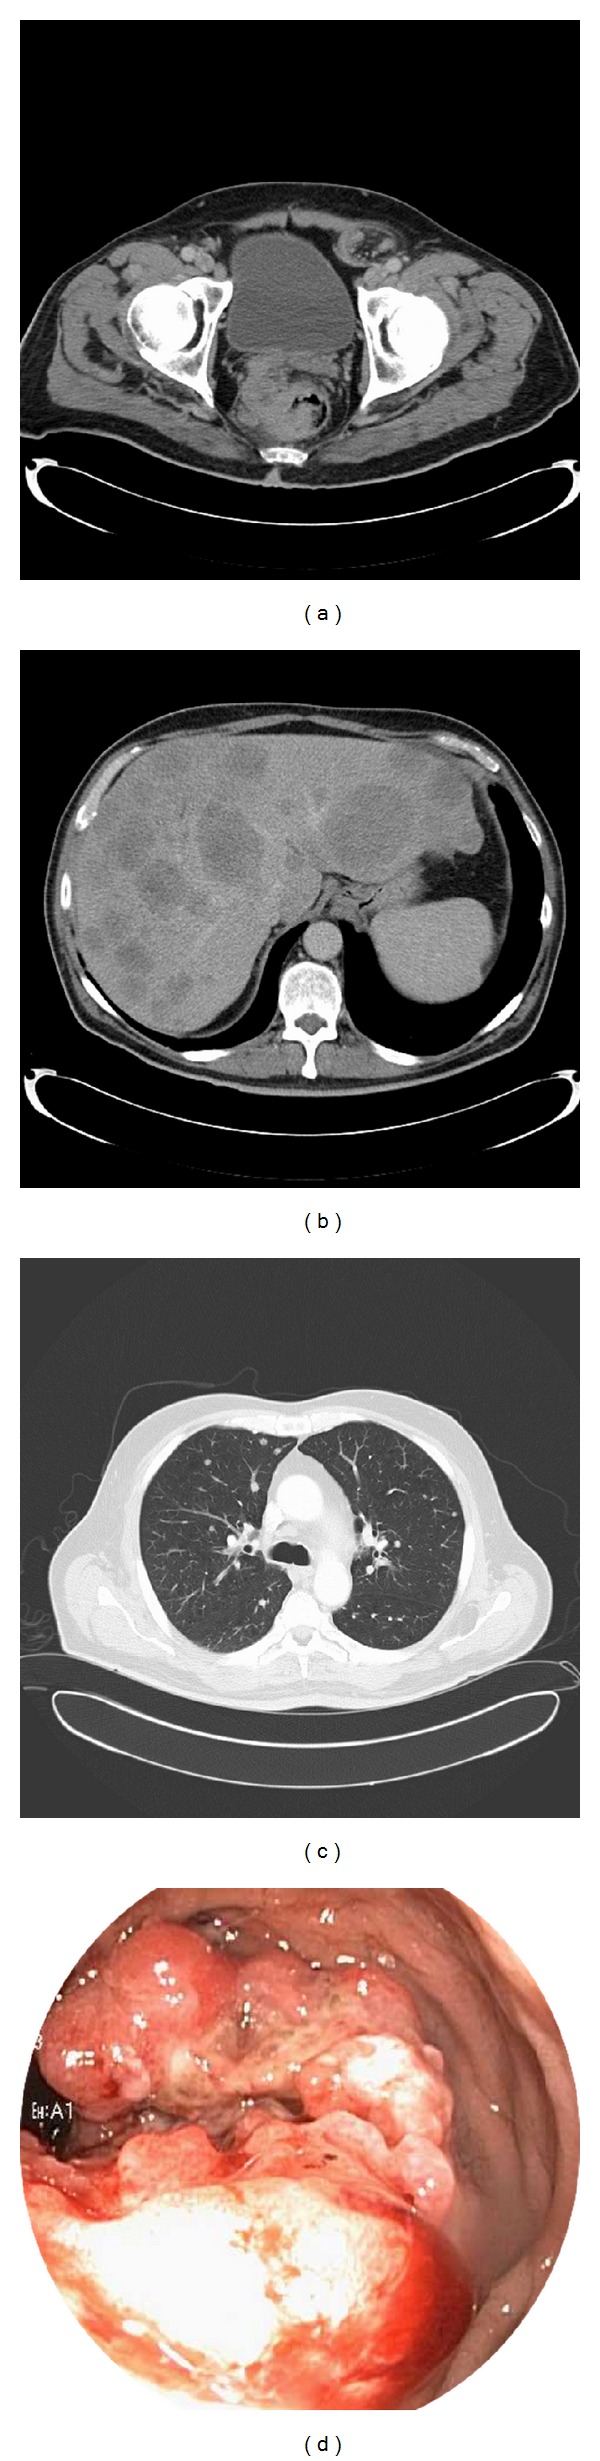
(a) Contrast-enhanced computed tomography scan of the pelvis reveals a rectal mass involving the anterior, right lateral, and posterior walls of the rectum. (b) Computed tomography scan showing numerous hypodense masses throughout the liver consistent with liver metastases. (c) Contrast-enhanced computed tomography scan of the chest showing bilateral lung nodules and mediastinal lymphadenopathy secondary to metastatic disease. (d) Picture from sigmoidoscopy showing a fungating, ulcerated, and partially obstructing rectal mass, involving two-thirds of the luminal circumference.
